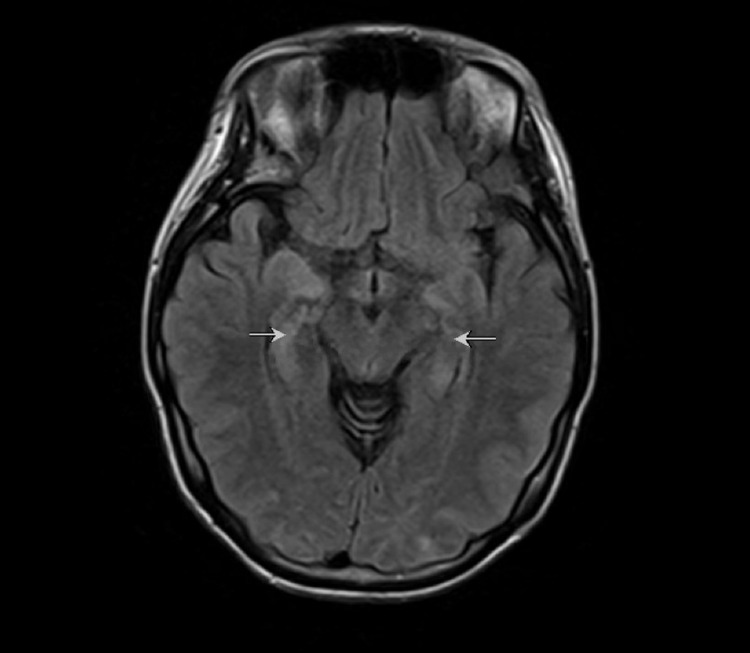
MRI brain with abnormal T2 signal in the bilateral medial temporal lobes (white arrows) concerning for autoimmune or infectious encephalitis. MRI: magnetic resonance imaging
